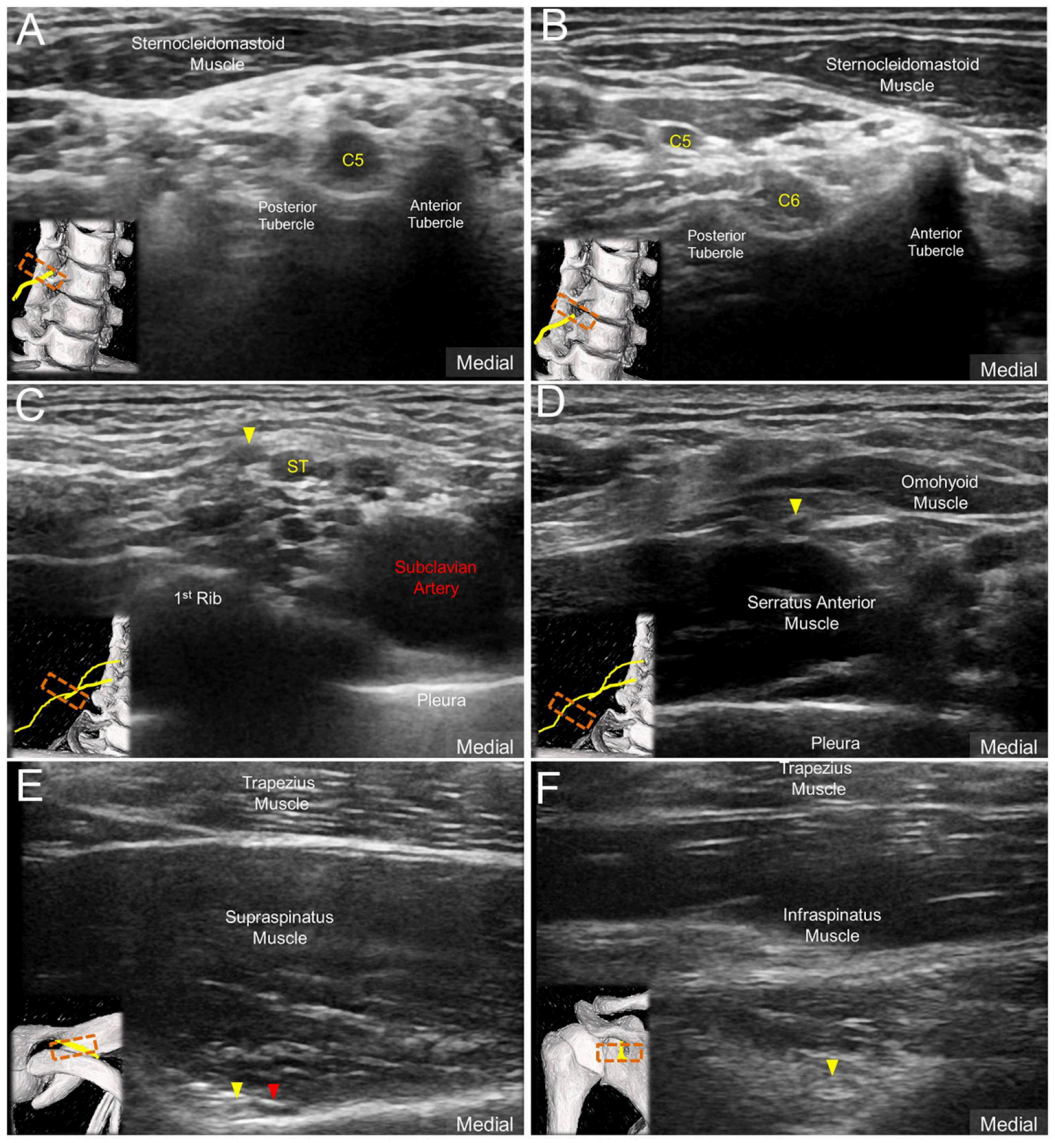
Ultrasound imaging of (A) C5 nerve root, (B) C6 nerve root and the suprascapular nerve *(solid yellow arrowhead)*
